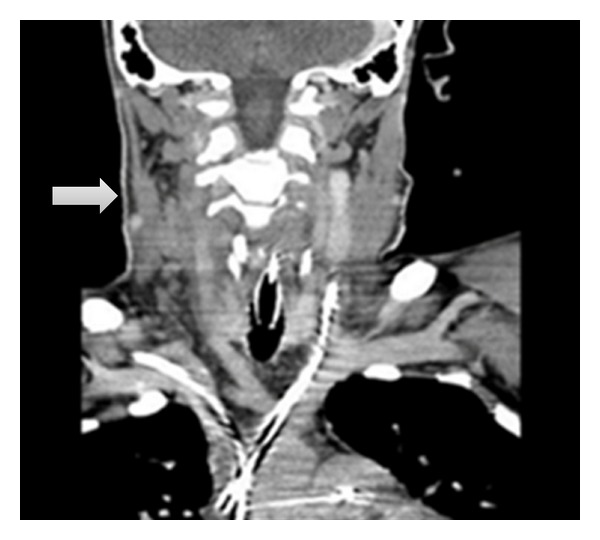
CT head shows a nonenhancing structure consistent wtih DVT at the right IJ vein (arrow).
